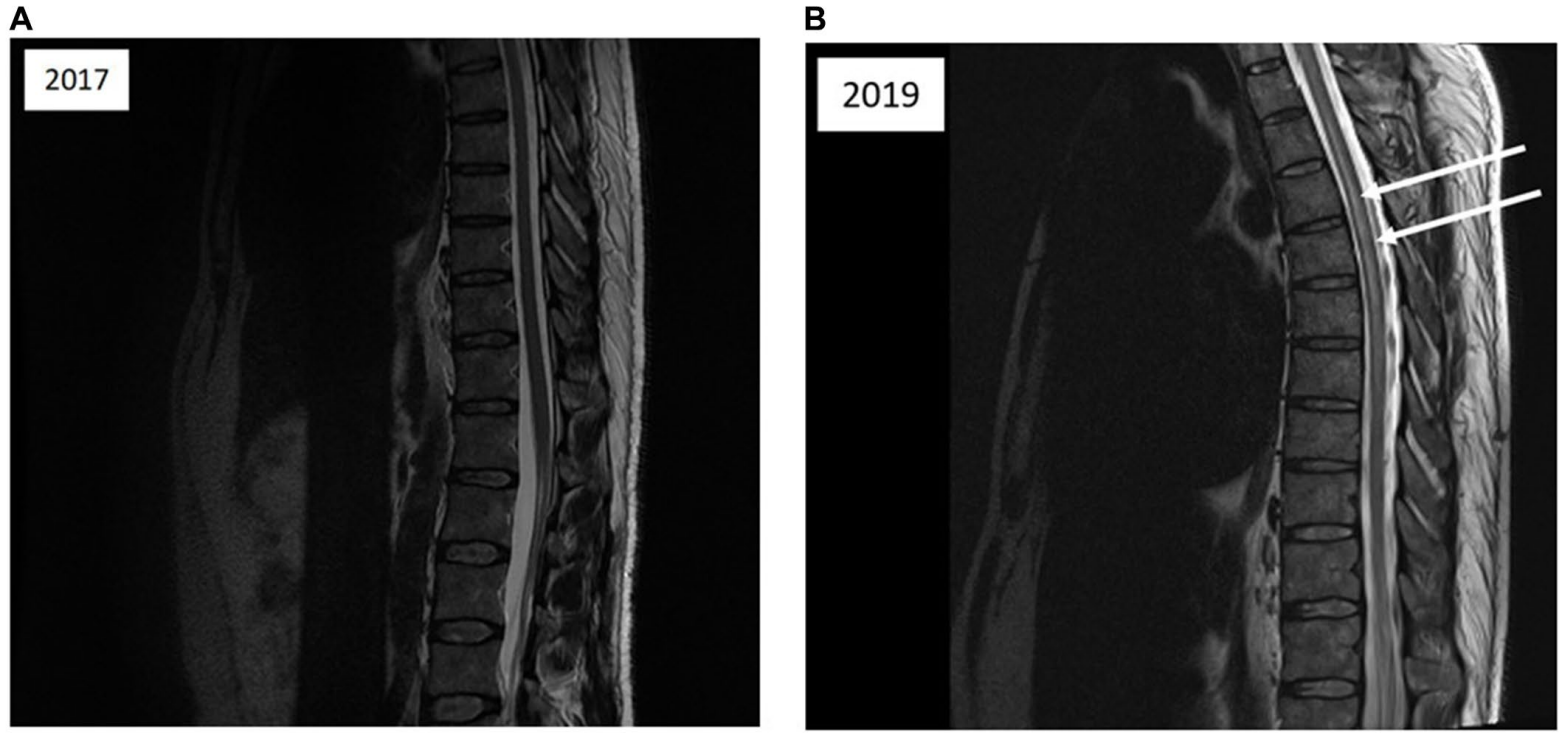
(A) Magnetic resonance imaging of the thoracolumbar spinal cord; sagittal T2-weighted images acquired 1 month after the initial diving incident reveal no abnormalities in spinal cord signal intensity. (B) Magnetic resonance imaging of the cervical spine; sagittal T2-weighted images acquired 1 month after the initial incident depict vertebral degenerative changes and medullary compression factors (indicated by arrows).
